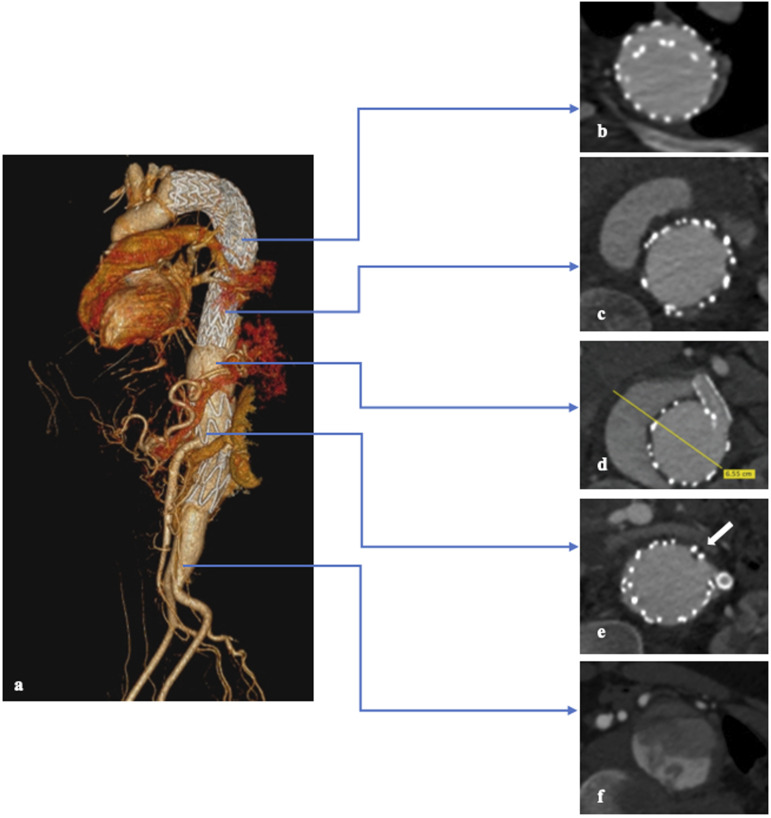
Preoperative CT scan of the patient after all the failed endovascular procedures. (a) TAAA 3D volume rendering after multiple endovascular procedures. (b) Axial view of overlapping of the thoracic endoprosthesis without any endoleak at this level. (c) Axial view showing the presence of type 2 Endoleaks with an increase of the diameter of the TAAA. (d) Axial view of the TAAA showing the largest diameter of the aneurysm, patent false lumen, and the CT stent. (e) Axial view that shows left renal stent and the reperfusion at this level (*white arrow*). (f) Dissection flap of the distal aorta (*red arrow*).
